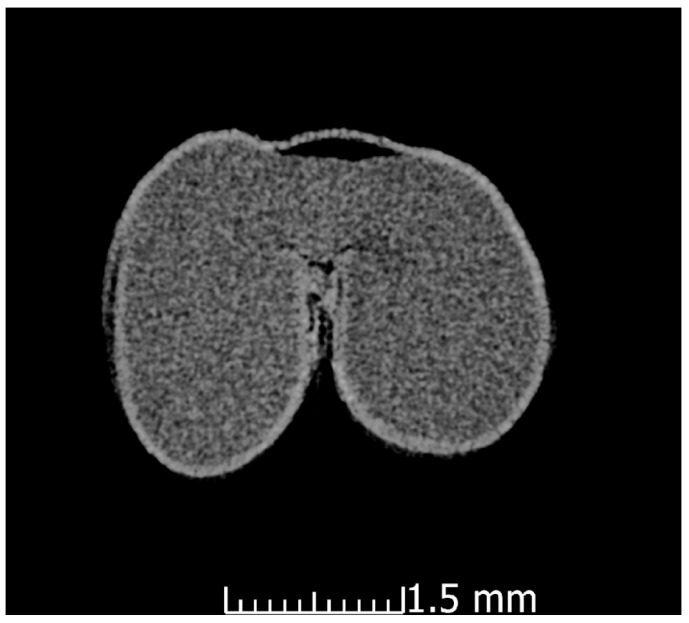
CT image of ‘Otilia’ cultivar wheat seed plasma-treated and swelling after PIXE measurement.
